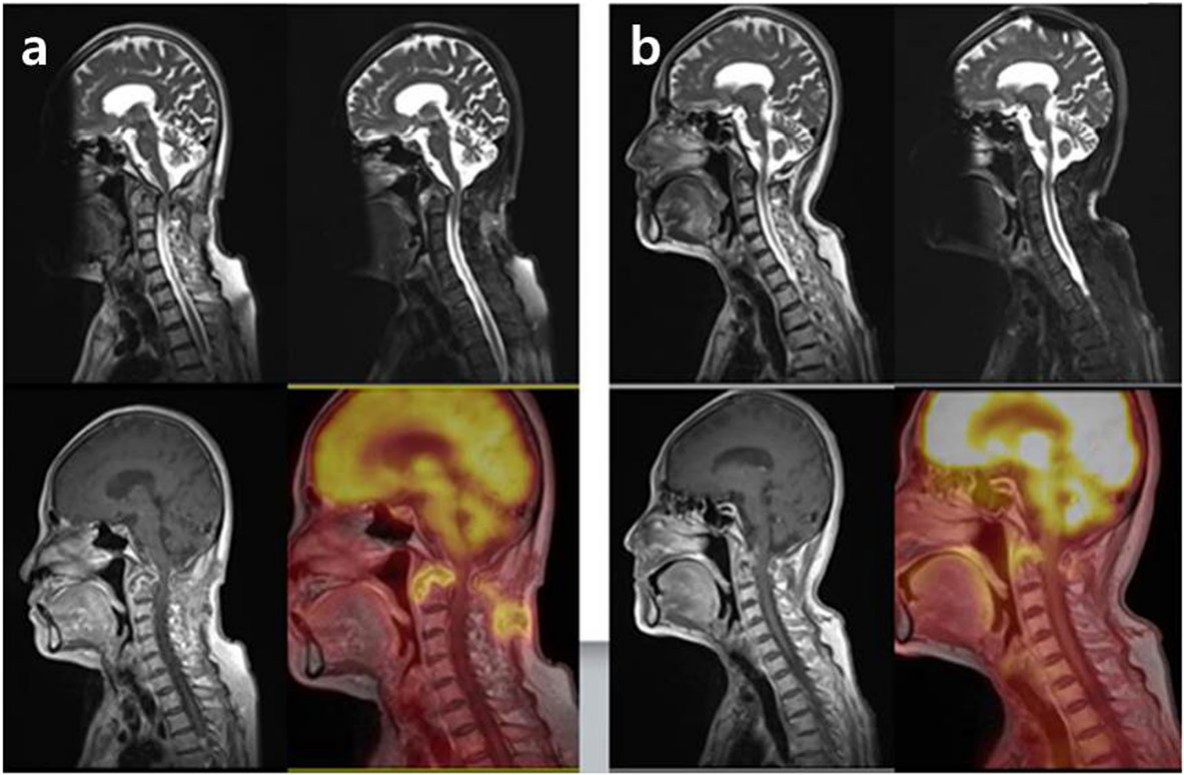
The second patient’s PET/MRI at 11.5-month anti-TB medication therapy (b) also revealed the decreased bone marrow edema with fatty change, pre- and intra-vertebral abscesses, and SUV_max_ (from 7.88 of PET/MRI at diagnosis to 4.14) on C1–2 compared with PET/MRI at diagnosis (a). However, positive AFB was detected from the granulation tissue of the surgical field, which resulted in additional anti-TB medication therapy
